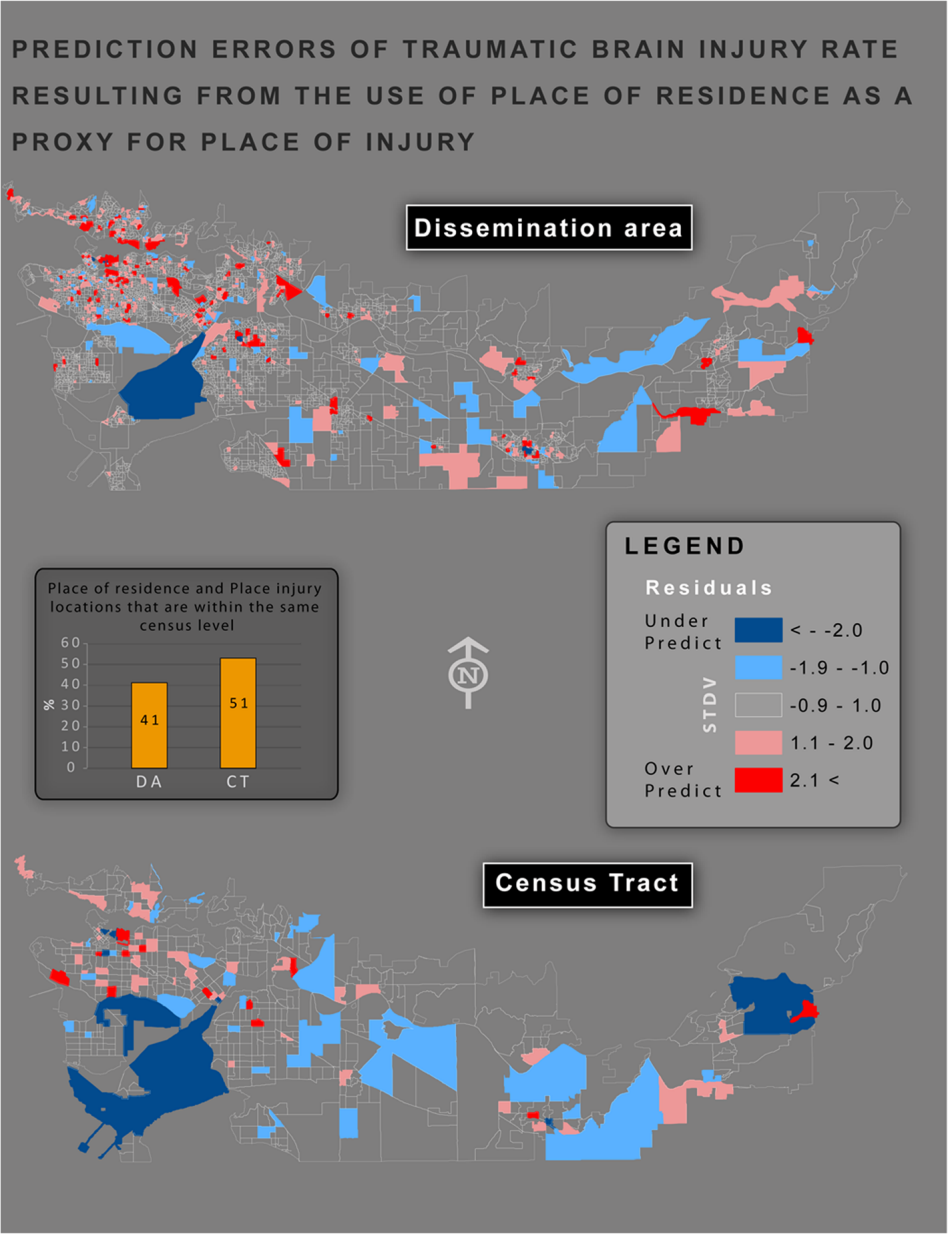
Shows the locational error at both the DA and CT level. The DA map clearly shows more detailed misclassification. This is because the DA’s smaller size makes it less common for both place of injury and place of residence to fall within the same area
